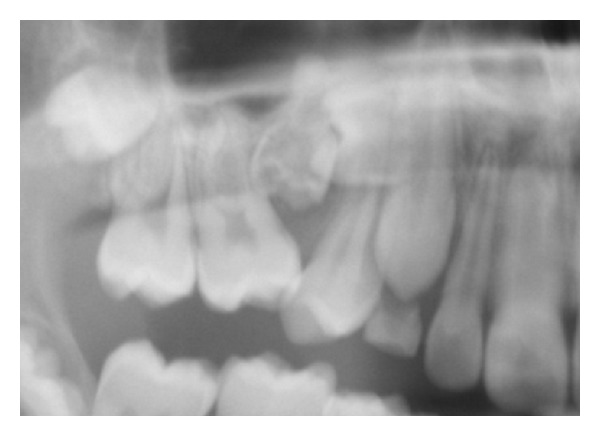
Section of a panoramic radiograph from a 14-year-old boy. The radiograph shows retention of the right primary second maxillary molar. The tooth has ankylosed and is not shed spontaneously. The tooth blocks normal development of the permanent dentition in the right side.
